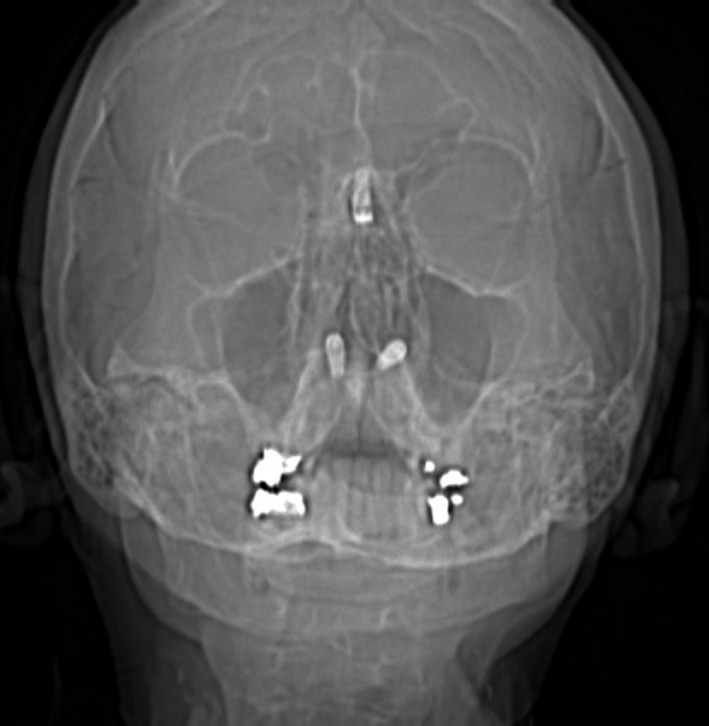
CT showing the three implants placed for prosthetic replacement support
